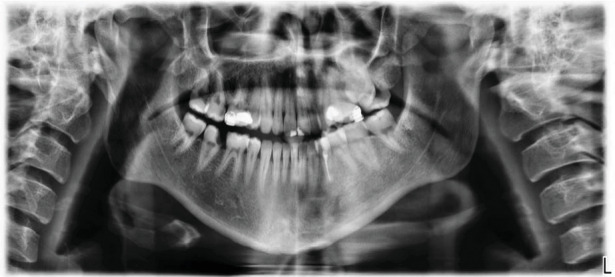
Panoramic radiograph of presenting patient confirms the lack of symmetry between single-rooted tooth #47 and contralateral tooth #37 which presents with two distinct roots.
